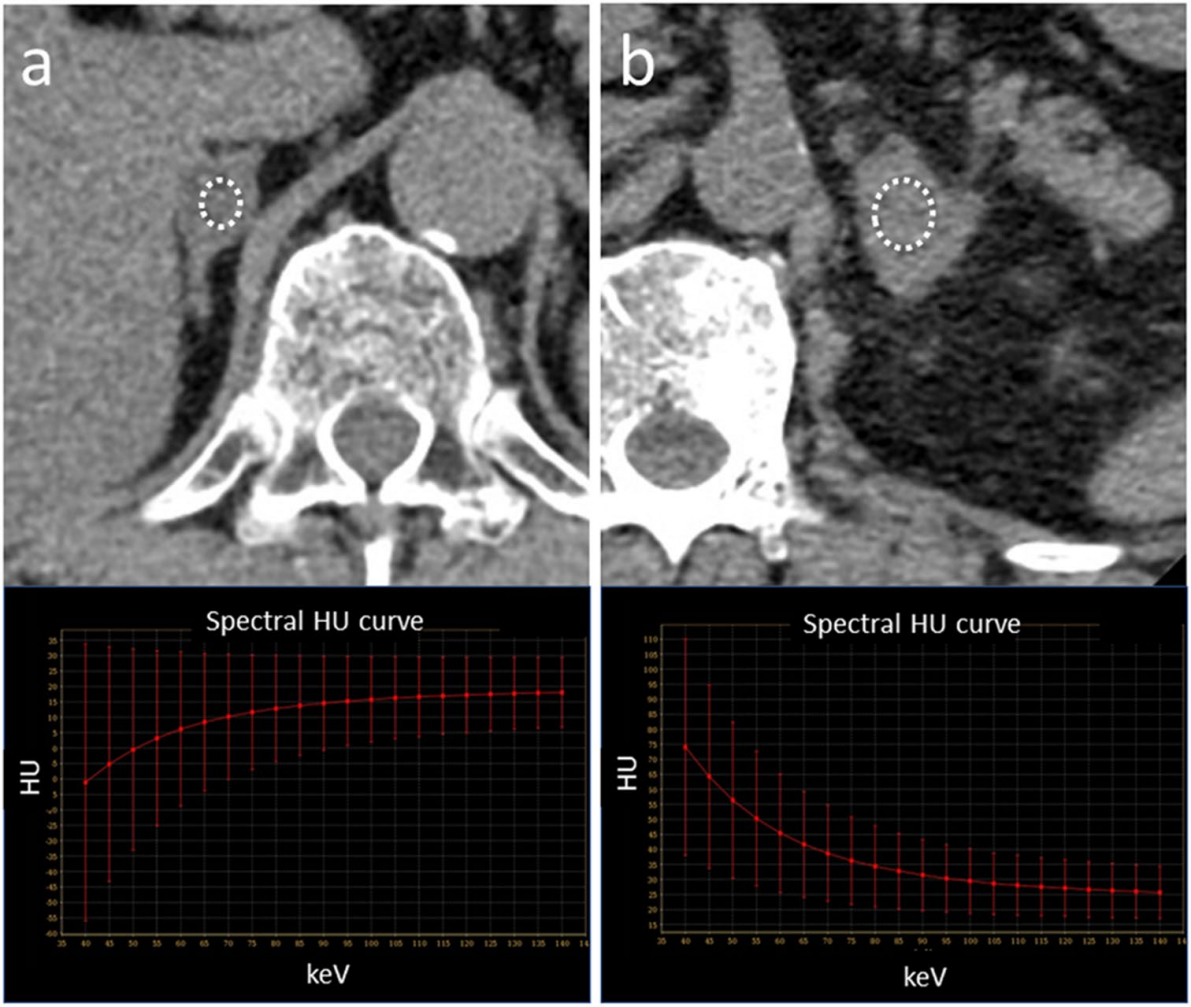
Axial monochromatic 70 keV images showing an adrenal adenoma (a) and an adrenal metastasis (b). Based on its CT number (HU = 19), the adenoma is not lipid-rich. At lower energy levels, the CT attenuation of the tumor decreases (a), suggesting that it contains fat. On the other hand, attenuation of the adrenal metastasis is increased at lower energy levels (b)
